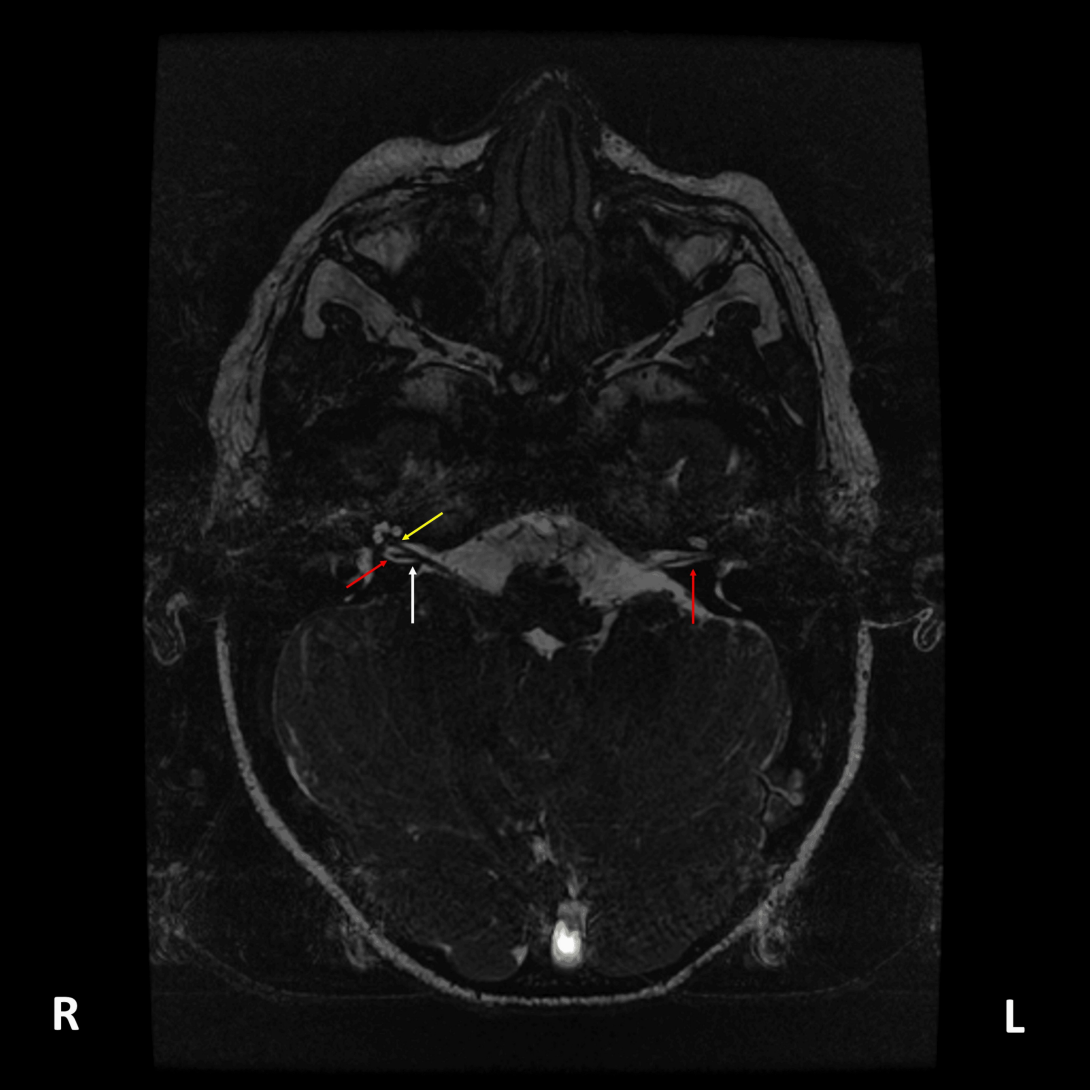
Axial FIESTA-C MRI image of the IAC. On the right (R) side, there is a clear separation of the vestibulocochlear nerve (white arrow) into the cochlear nerve anteriorly (yellow arrow) and the vestibular nerve posteriorly (red arrow). On the left (L) side, this separation of the vestibulocochlear nerve is not observed, and only the vestibular nerve is visualized (red arrow), showing apparent thinning compared with the contralateral side. FIESTA-C: fast imaging employing steady-state acquisition - C-variant; MRI: magnetic resonance imaging; IAC: internal auditory canal; R: right side; L: left side.
